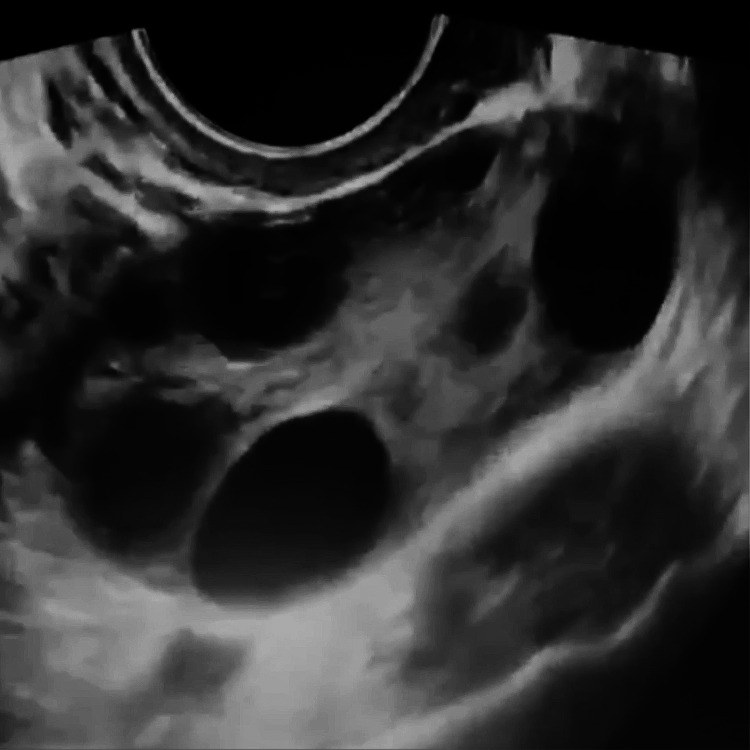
Transvaginal ultrasound shows an ovary with multiple cysts
